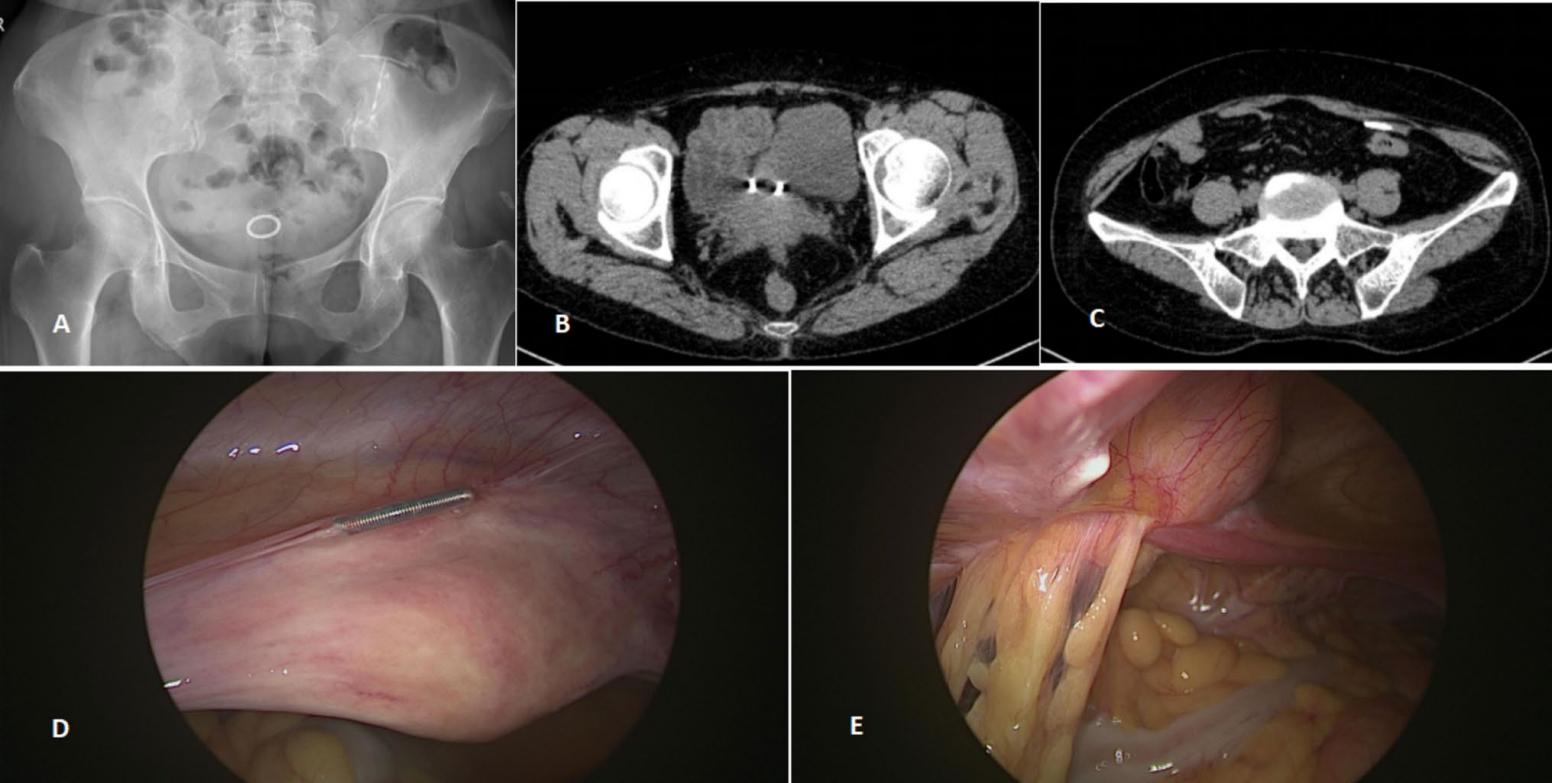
A X-ray showed an IUD in the pelvic cavity and another in the left iliac fossa. B and C Pelvic CT showed a circular IUD in the interspace between the bladder and the uterus and a T-shaped IUD in the left iliac fossa. D and E An IUD was seen between the anterior uterine wall and the bladder. Another was seen on the left anterior abdominal wall
